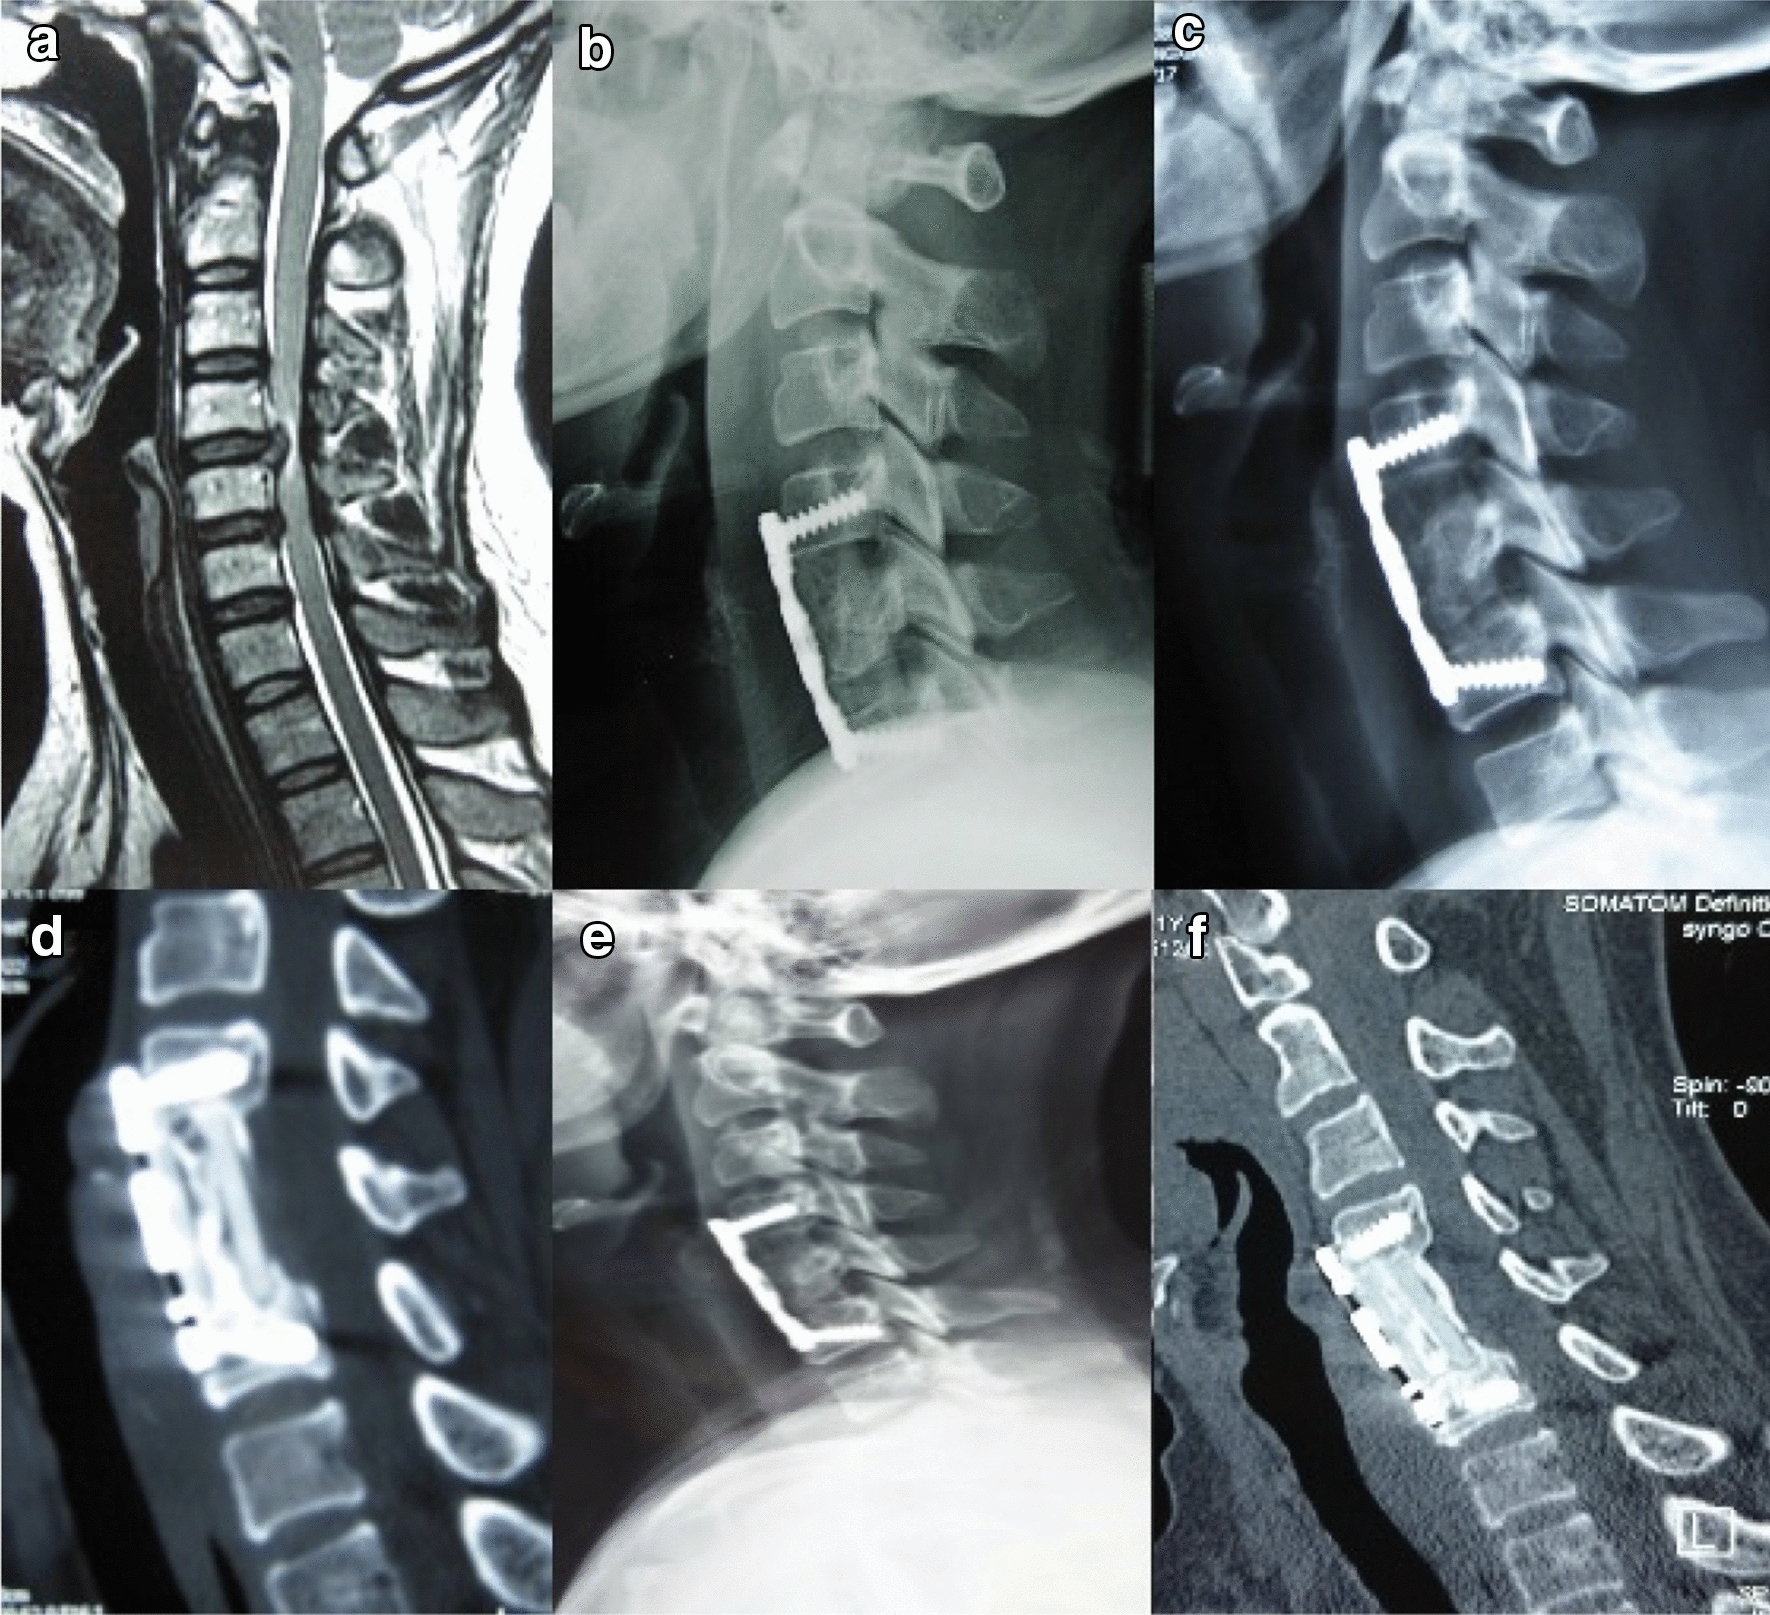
Type b bone fusion. a–f A 41-year-old woman whose preoperative cervical MRI (a) revealed C4/5 disc herniation compressing the spinal cord underwent 1-level corpectomy with an n-HA/PA66 strut. Lateral X-ray films indicated bony fusion of the autogenous bone granules filling the strut, and subsidence was observed at the 6-month, 12-month, and 8-year follow-up visits (b, c, e). 3-D CT at the 3-year and 8-year follow-up visits (d, f) showed solid bone fusion
